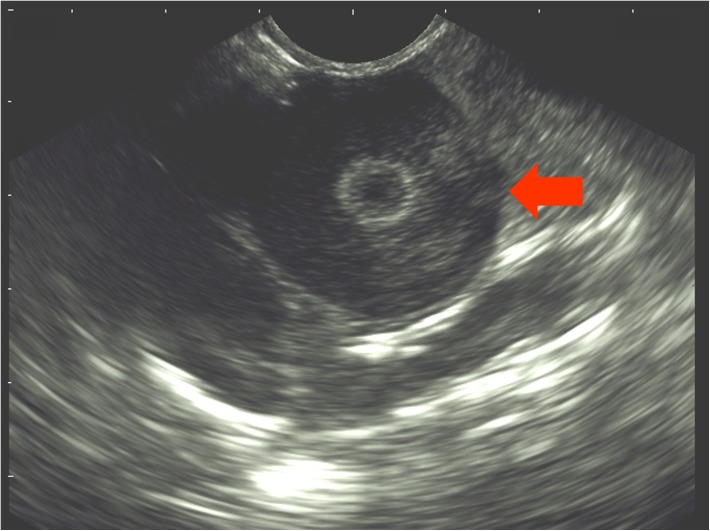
Sonogram of the ovary of a hen. The arrow marks an ovarian follicle.
